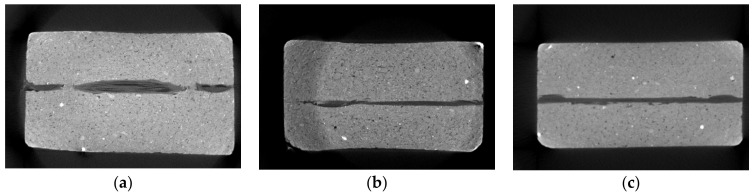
(a) CT scan of carbon textile with Mortar 1, (b) CT scan of carbon textile with Mortar 2, (c) CT scan of carbon textile with Mortar 3.
